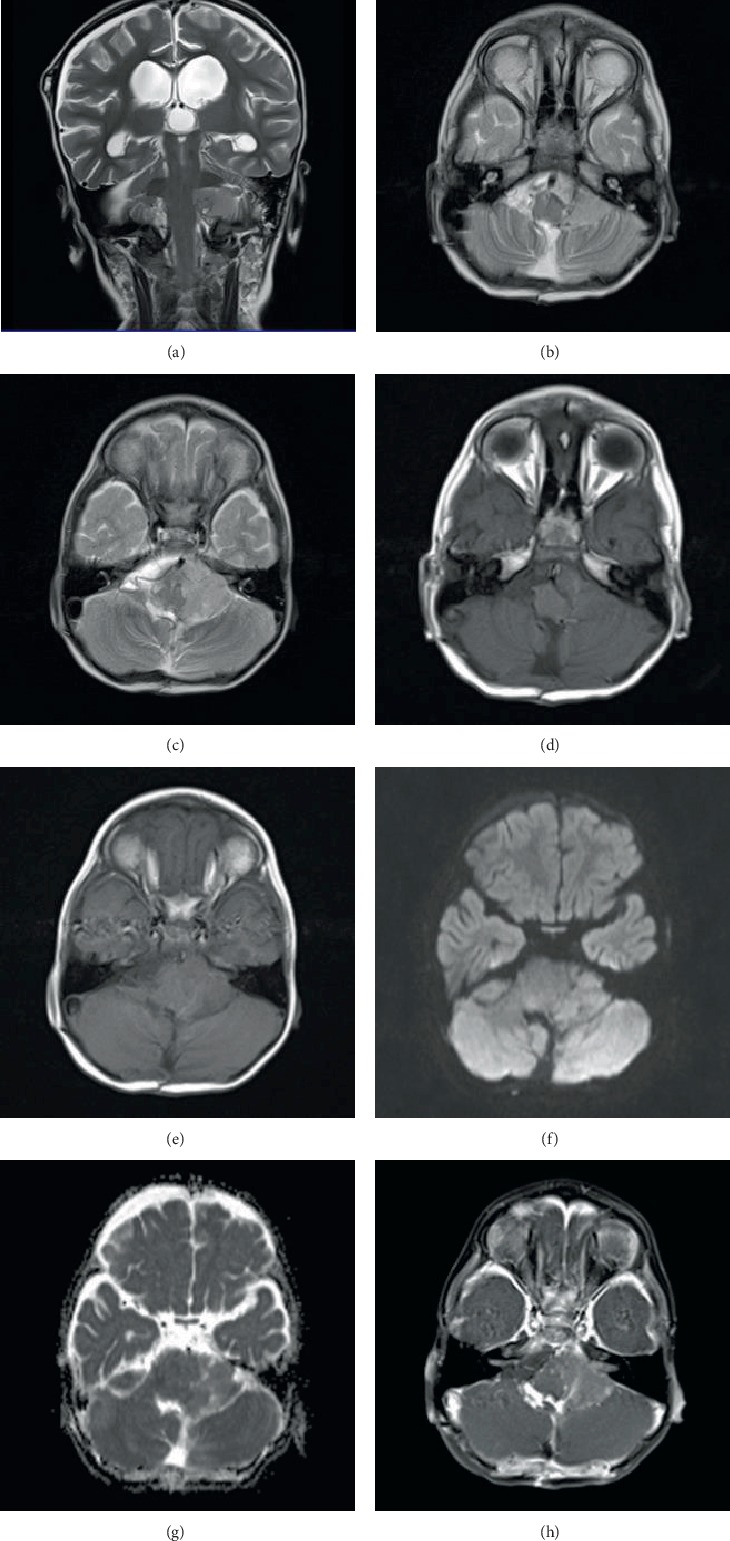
(a) Coronal T2 WI. (b, c) Axial T2 WI. (d, e) Axial T1 WI. (f, g) Axial DWI and ADC map. (h) Axial T1 postcontrast. (a–h) A large soft tissue mass is noted at the left cerebellopontine angle demonstrating an intermediate hyperintense signal on T2WI and hypointense signal on T1WI with mild restriction on DWI and faint homogenous enhancement after contrast. (a) The mass is causing hydrocephalus which has resolved with bilateral small subdural collections, related to the reduced intracranial pressure, following surgery.
